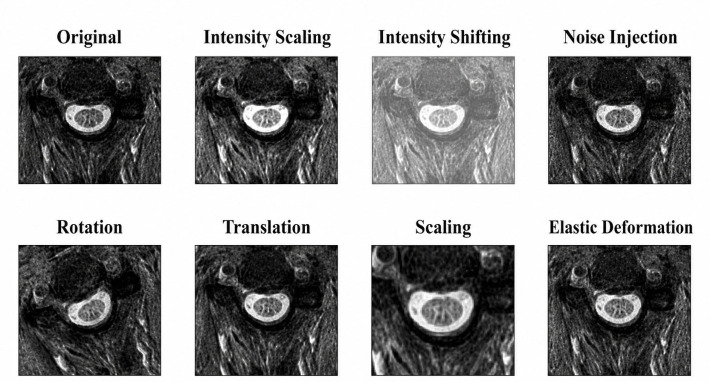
Examples of data augmentation techniques applied to an SC MRI image.
